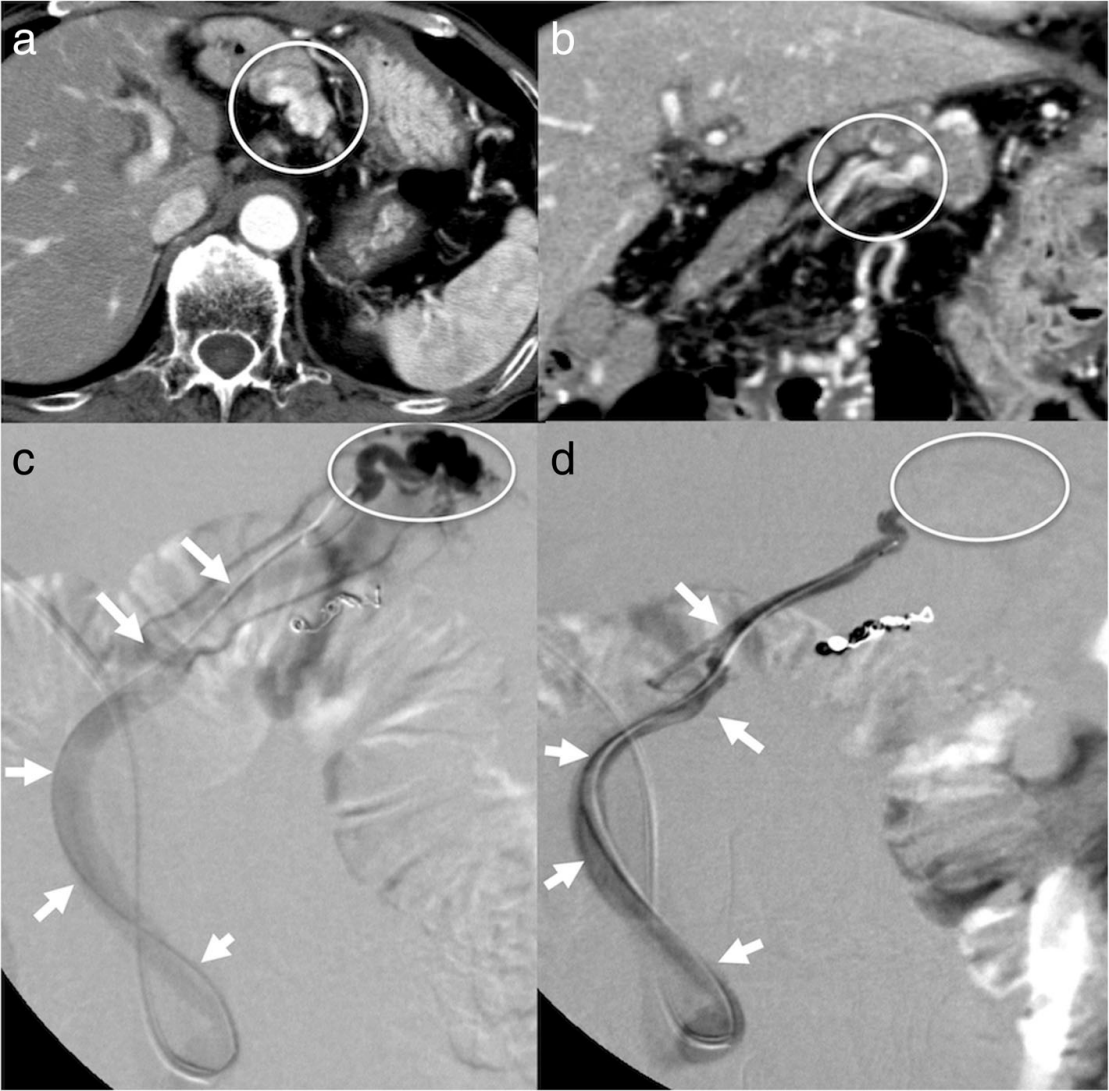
a, b Computed tomography image showing varices at the pancreaticojejunostomy that communicated with the elevated jejunal and portal veins. c, d Image of retrograde trans-portal-venous obliteration (white arrow is the elevated jejunal vein). Retrograde venography before obliteration (c) showing the varices around the pancreaticojejunostomy (white circle). Retrograde venography after obliteration (d) showing that the varices around the pancreaticojejunostomy disappear (white circle)
